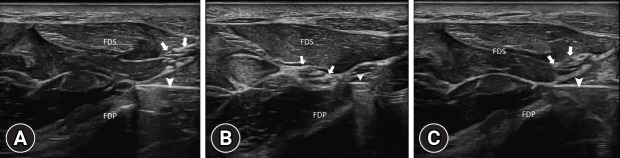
Short-axis dynamic ultrasound examination at the forearm level demonstrates the median nerve (arrows) as follows: (A) away from the screw (arrowhead), (B) near the screw, and (C) subsequently moving away from the screw. FDS, flexor digitorum superficialis; FDP, flexor digitorum profundus.
